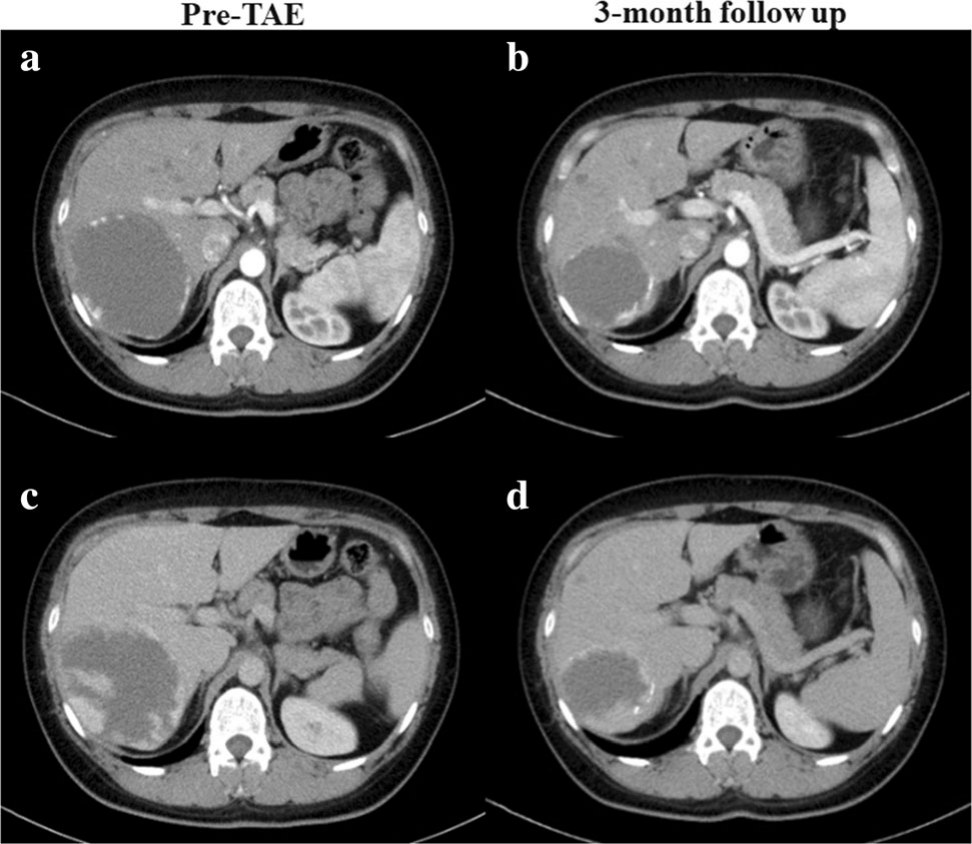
A 39-year-old woman with a 11 cm HH. CT in the arterial phase A and venous phase C showed a large hepatic hemangioma in the right liver. Three months after TAE treatment, the patient received the first re-examination and CT in the arterial phase B and venous phase D revealed the volume of the hemangioma was decreased
